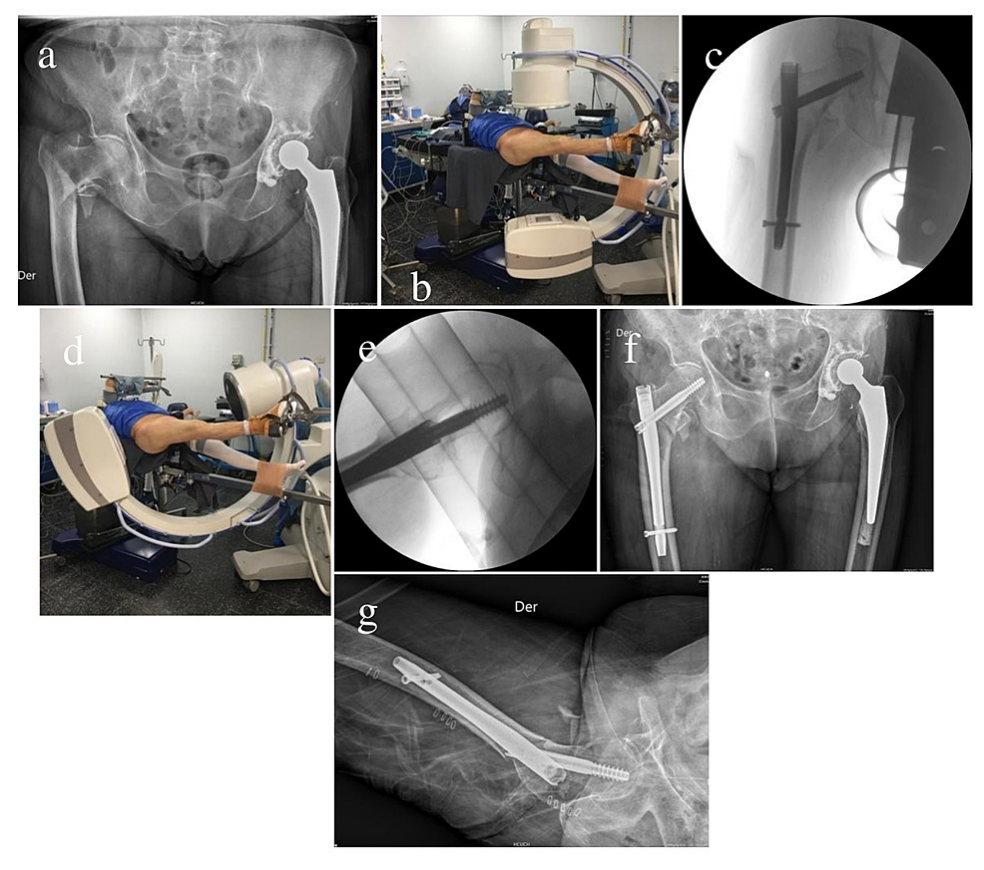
Left total hip arthroplasty and right intertrochanteric fracture (a) For an 85-year-old female patient, the anteroposterior (AP) radiograph shows that left total hip arthroplasty had previously been performed and there was a right intertrochanteric fracture. (b) The well-leg scissor position on the fracture table and C-arm in the AP position. (c) AP fluoroscope of the cephalous nail system after fracture reduction. (d) Well-leg scissor position and C-arm in the axial view. (e) Axial fluoroscope view of the cephalous nail system after fracture reduction. (f and g) Immediate postoperative pelvis X-ray in the AP and axial views.
